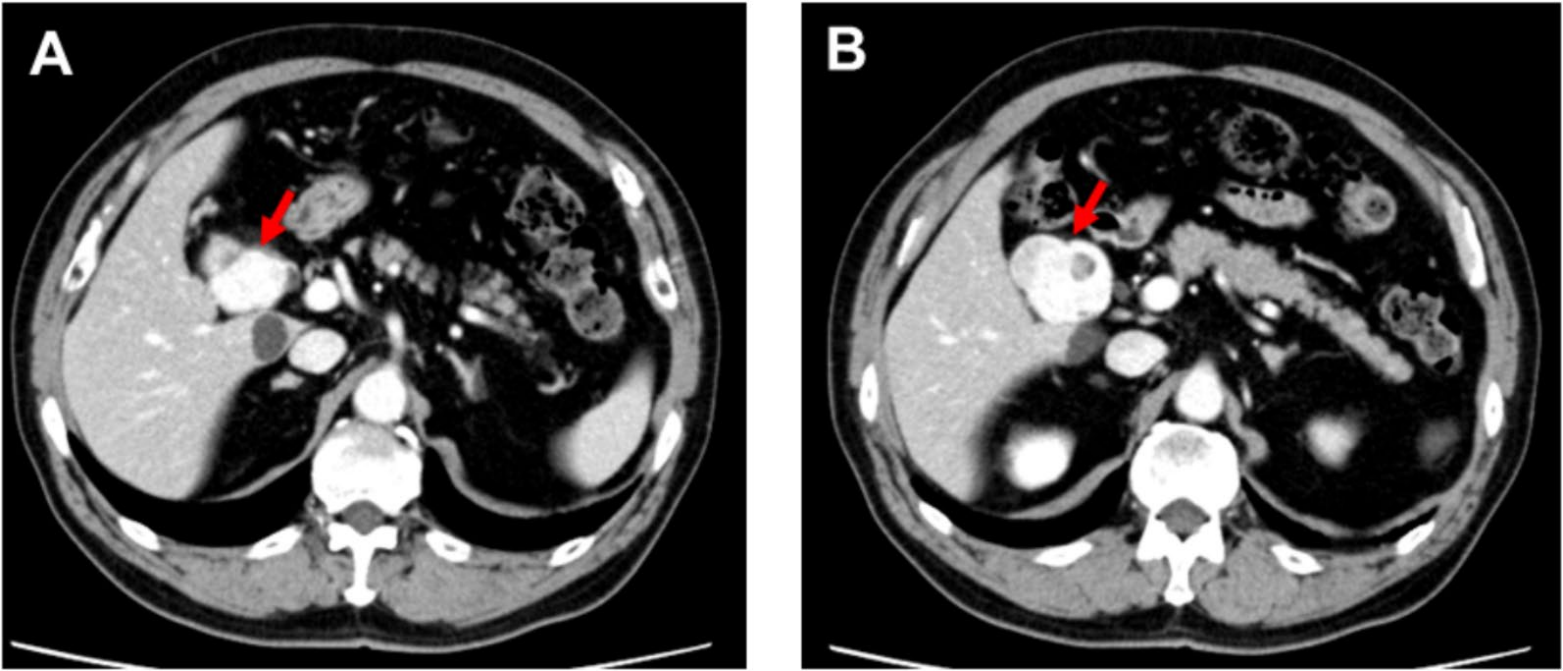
Abdominal contrast-enhanced computed tomography imaging. A A mass measuring 48 mm in diameter on the right side of the common bile duct (arrow). B The border of the mass was clear with no obvious invasion (arrow)
